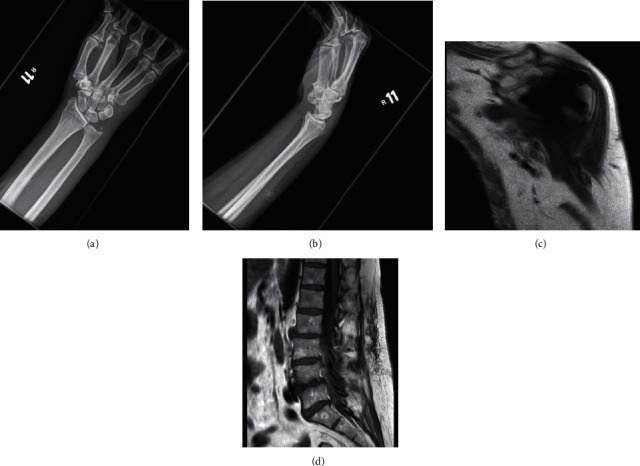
(a) PA X-ray right wrist, (b) lateral X-ray right wrist, (c) T1 coronal MRI right shoulder, and (d) T1 sagittal lumbar spine.
